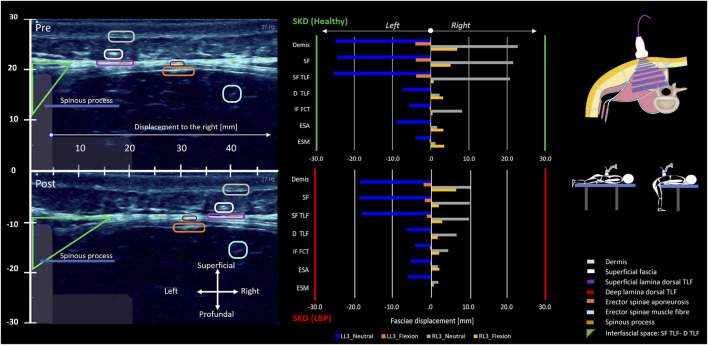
Lumbodorsal SKD-induced stress transmission to underlying tissues and their displacements and deformations. The two individuals, healthy (green y-axis) and LBP (red y-axis), were placed prone on a physio plinth. A foam block under the SIAS obtained 10° lumbosacral flexion, the neutral position (blue and grey). For the flexed position, subjects were positioned at the end of the physio plinth with legs on the floor and forearms on the plinth to maintain 60° spinal flexion (orange and yellow). Ultrasonography videos were recorded in both neutral and flexed positions. Post-analysis of the ultrasonography recordings was performed using speckle tracking analysis. With the obtained data, the absolute displacement in millimeters (mm) of each anatomical structure (epidermis, superficial fascia, TLF, ESA, ESM, SPI, LD) was calculated. Additionally, the shear strain ratio between the (epi)dermis and each anatomical structure was calculated. In the ultrasonography images, the region of interest for each anatomical structure is circled and corresponds to the colors in the legend. The images represent the absolute displacements of anatomical landmarks in mm due to the SKD maneuver to the right and left at location L3. The region of interest circles allow for visual estimation of the absolute displacement. The plots show the displacement results in mm in neutral (blue and grey) and flexed positions (orange and yellow). The absolute displacement of the anatomical structures is greatest superficially and decreases in depth. Shear strain ratios between the (epi)dermis, superficial fascia, and the TLF were small, indicating equal displacement. In contrast, higher ratios between the (epi)dermis and back muscles suggested a greater shear strain ratio and potentially higher shear stress (see Tables 1, 2). Abbreviations: SKD, skin displacement; SF, superficial fascia; D TLF, deep thoracolumbar fascia; IF FCT, interfascial fat; SF TLF, superficial thoracolumbar fascia; ESA, erector spinae aponeurosis; ESM, erector spinae muscle.
